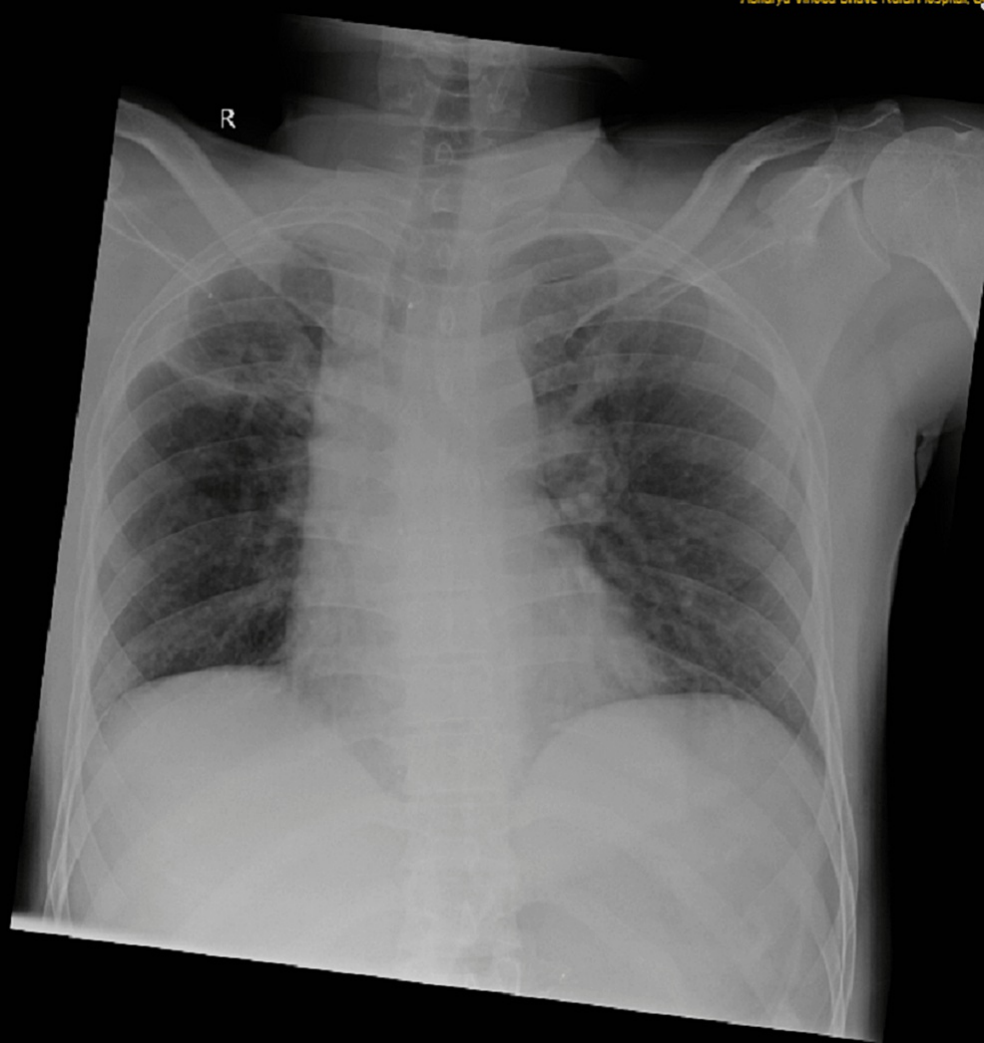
Radiograph of the chest showing no injury
